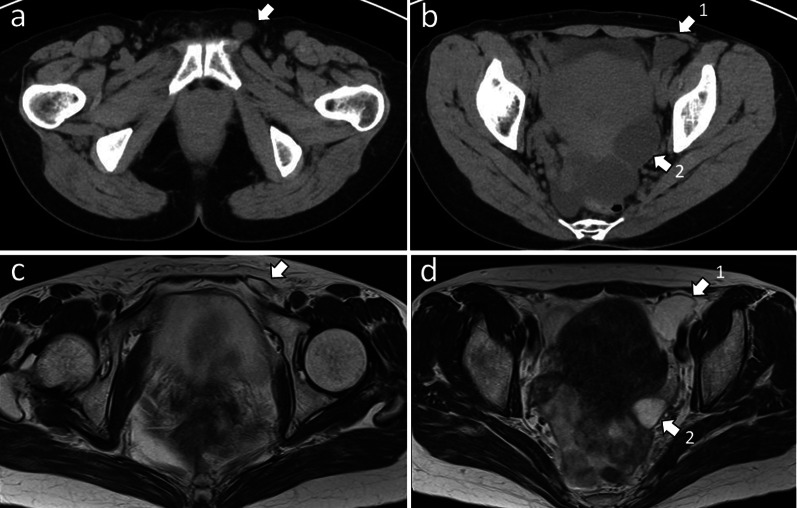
Computed tomography scan and magnetic resonance imaging findings of a hydrocele of the canal of Nuck extending from the abdominal cavity to the subcutaneous. a Computed tomography scan findings. Arrow: subcutaneous hydrocele. b Computed tomography scan findings. Arrow 1: hydrocele protruding into the abdominal cavity. Arrow 2: ovarian cyst. c. T2-weighted magnetic resonance imaging finding. Arrow: hydrocele in the inguinal canal. d. T2-weighted magnetic resonance imaging finding. Arrow 1: hydrocele protruding into the abdominal cavity. Arrow 2: ovarian cyst
